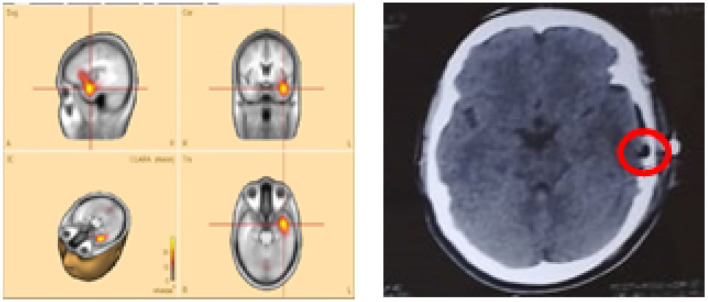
Sample patient no. 8. (Left) the CLARA area, and (right) the resected area in the post-surgical CT-scan.
